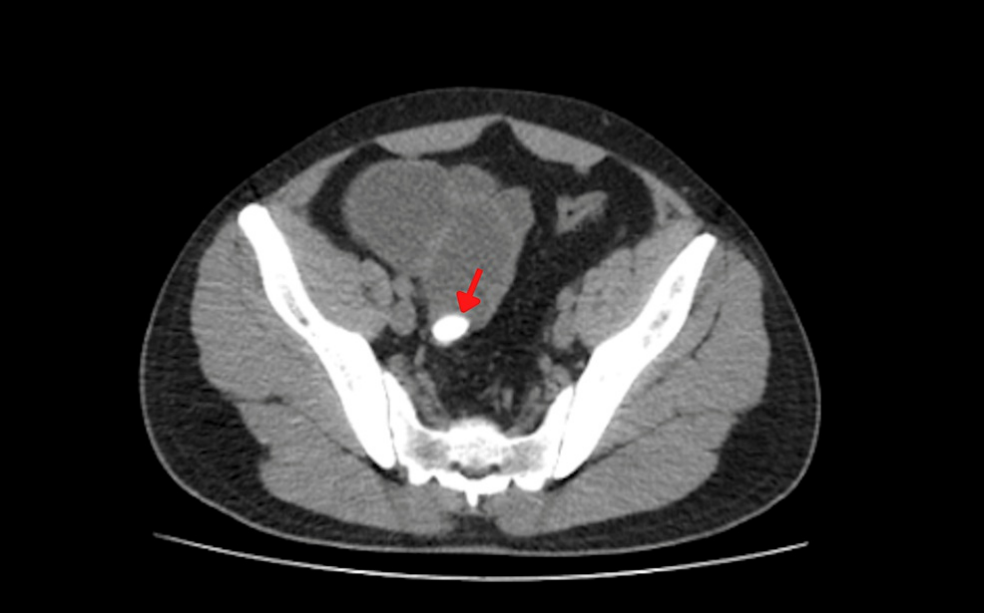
Axial view of abdominal computed tomography showing the gallstone in the distal ileum (the red arrow).
